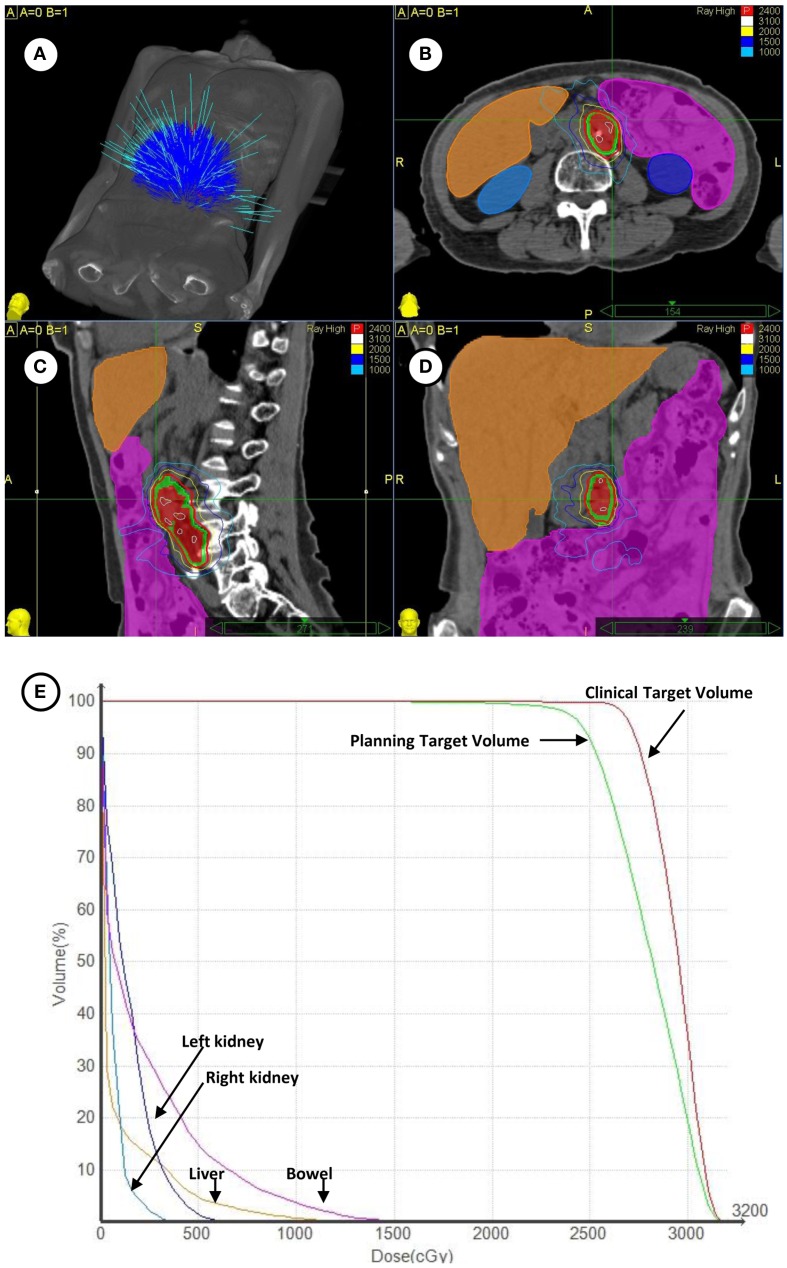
(A) Depicted are the 140 treatment beams by the Cyberknife radiosurgery 6 MV accelerator for treatment during the targeting of left-sided para-aortic lymph nodes. (B–D) Depicted are axial, coronal, and sagittal projections of radiosurgical treatment. The ^18^F-FDG PET/CT-derived clinical target volume (red shaded volume) and 3 mm expanded planning tumor volume (green shaded volume) are contoured. The 24 Gy prescription isodose is highlighted in red and a 10 Gy isodose is outlined in light blue. Contouring of the bowel (magenta), liver (orange), right kidney (light blue), and left kidney (dark blue) is shown. (E) Plotted are corresponding radiation dose-volume histograms for clinical targets and organs at-risk.
